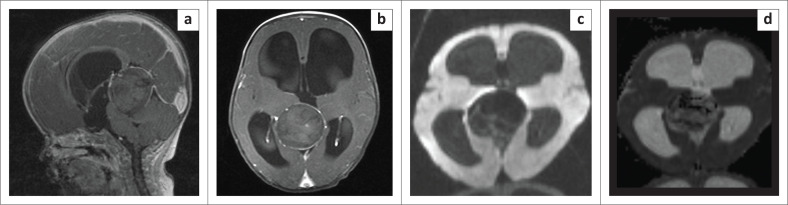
Follow-up MRI on day 3 post-procedure: (a) T1-fat-saturated post-contrast mid-sagittal, (b) T1-fat-saturated post-contrast axial, (c) Diffusion-weighted imaging axial at b 1000 s/mm^2^ and (d) Apparent diffusion coefficient axial at the same level as (c); showing the vein of Galen aneurysmal malformation thrombosed sac with hydrocephalus, mild peripheral rim enhancement and absence of restricted diffusion.
